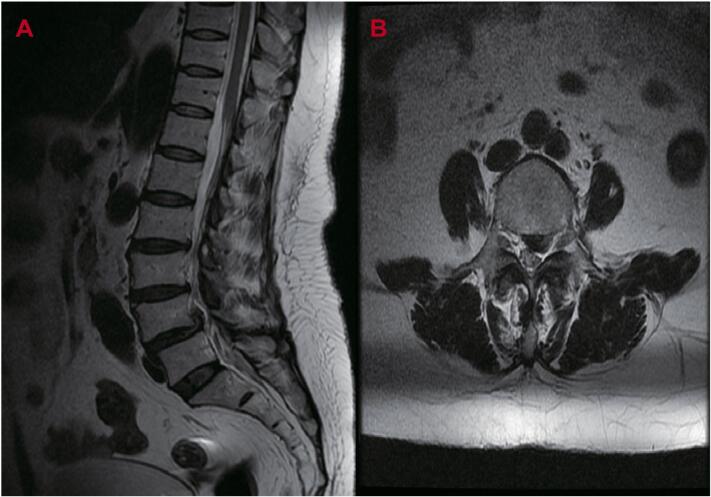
A-B. Preoperative Sagittal (A) and axial (B) MR images revealed intervertebral disc extrusion at the L4/5 segments towards the posterior and superior aspects, concurrent with spinal canal stenosis and left lateral recess stenosis.
